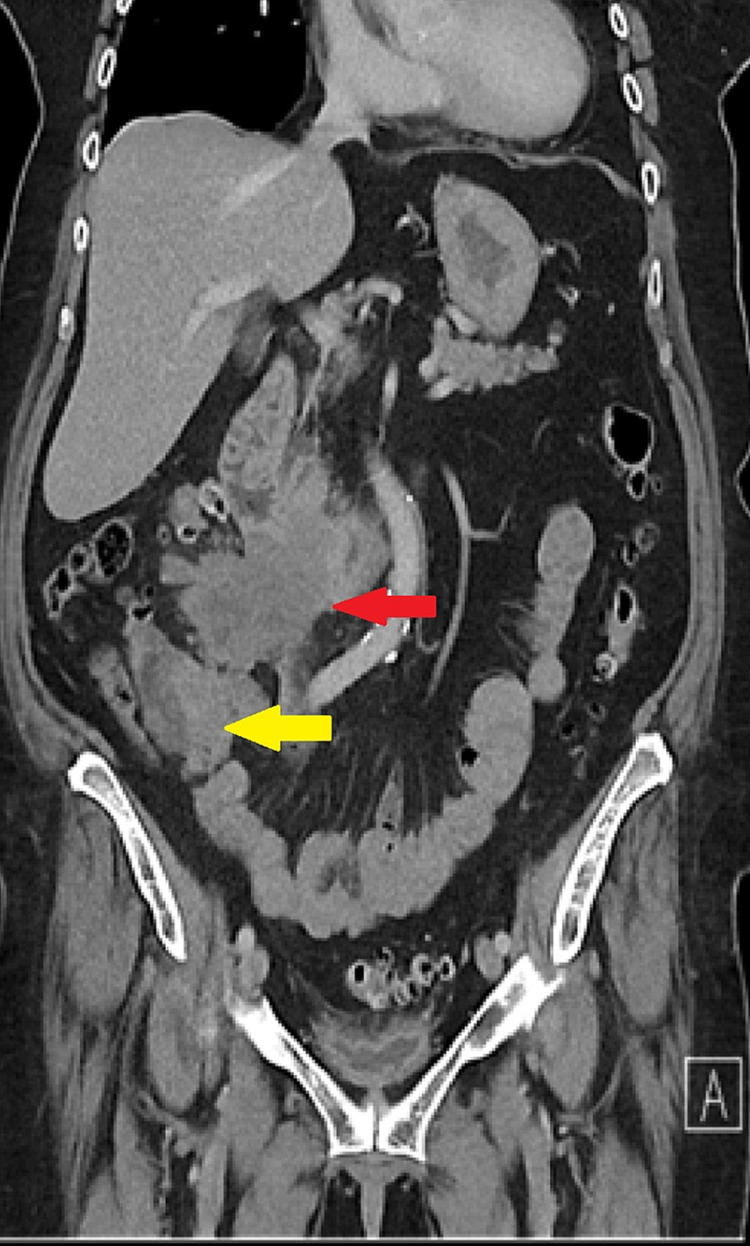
CT scan showing thickened D3 segment leading to a large irregular mass (red arrow). This is closely associated with the primary caecal mass (yellow arrow).
